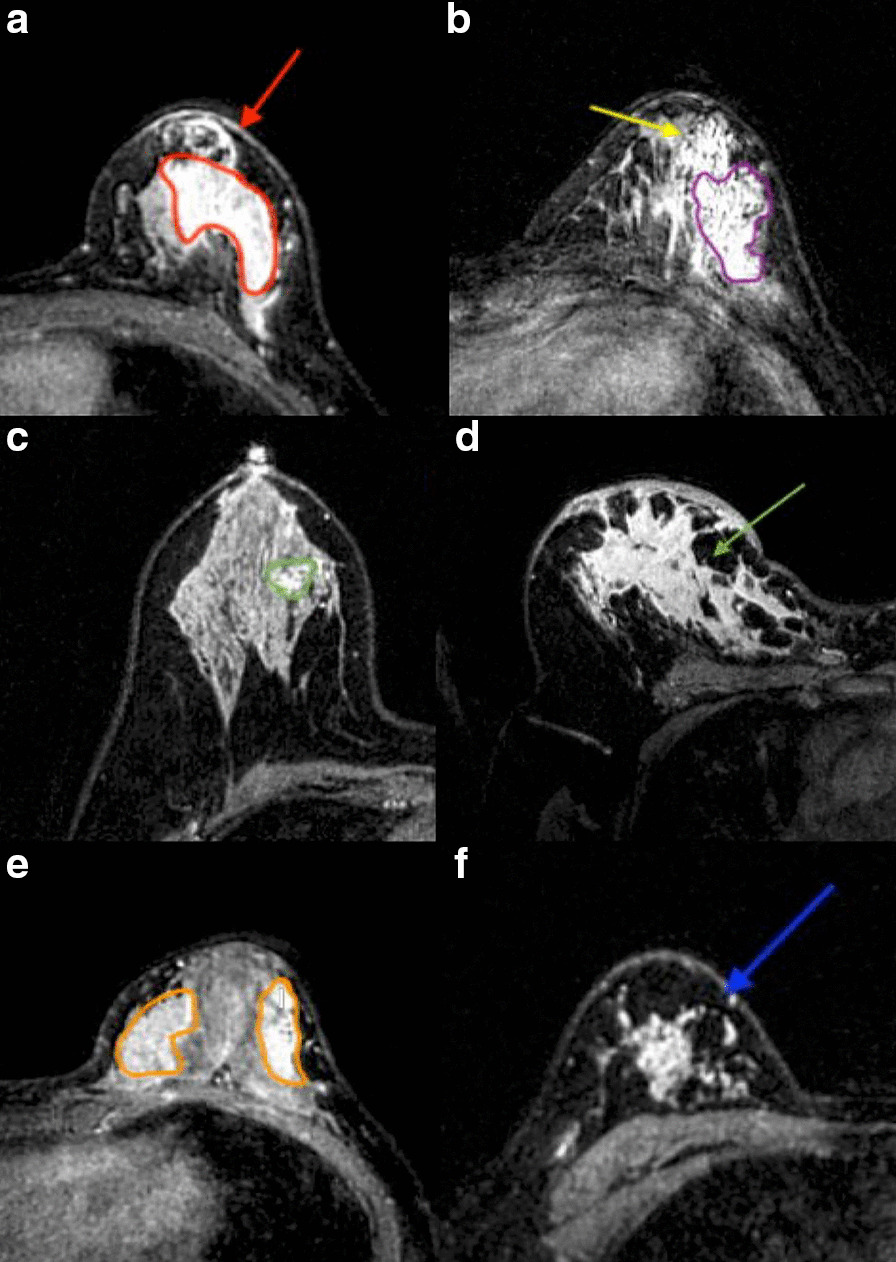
The sequence of dynamic imaging performed using axial T1-weighted fat-suppression. a The second contrast-phase image shows an NME with homogeneous internal enhancement patterns (red irregular shape) and a linear distribution (red arrow). b The third contrast-phase image shows that the entire lesion enhancement was heterogeneous, with intermingling local, cluster-ring, and clumped enhancement (purple irregular shapes) and the appearance of segmental distribution (yellow arrow). c, d respectively show the focal (green irregular shape) and diffuse (green arrow) distribution patterns of NME in the fifth contrast-phase image. e, f Respectively show the regional (orange irregular shape) and multiple-region (blue arrow) distributions in the second contrast phase
